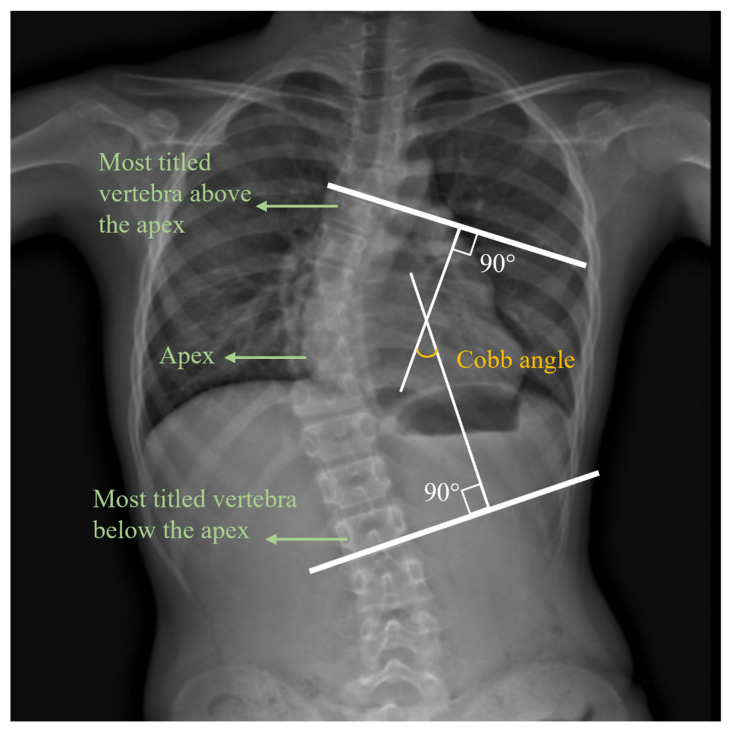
An X-ray image of the Cobb angle.
